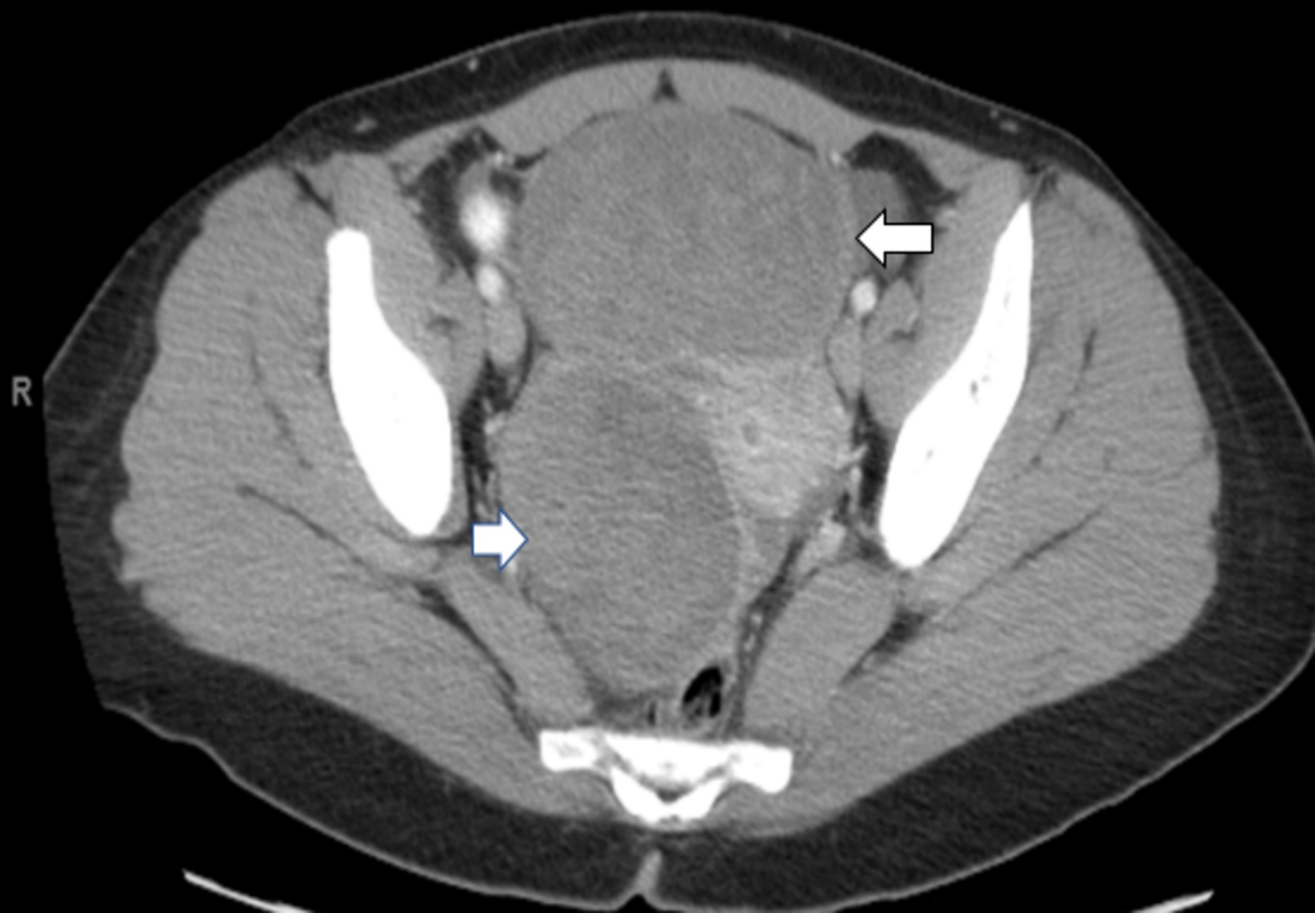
Case 2: imaging of the pelvis Magnetic resonance imaging of the pelvis was obtained six weeks after her initial positron emission tomography/computed tomography to evaluate the areas of hypermetabolic activity. This showed metastases to bilateral ovaries. The anterior mass was 9.2 x 7.9 x 9.1 cm and the posterior mass was 6.3 x 5.6 x 5.6 cm.
